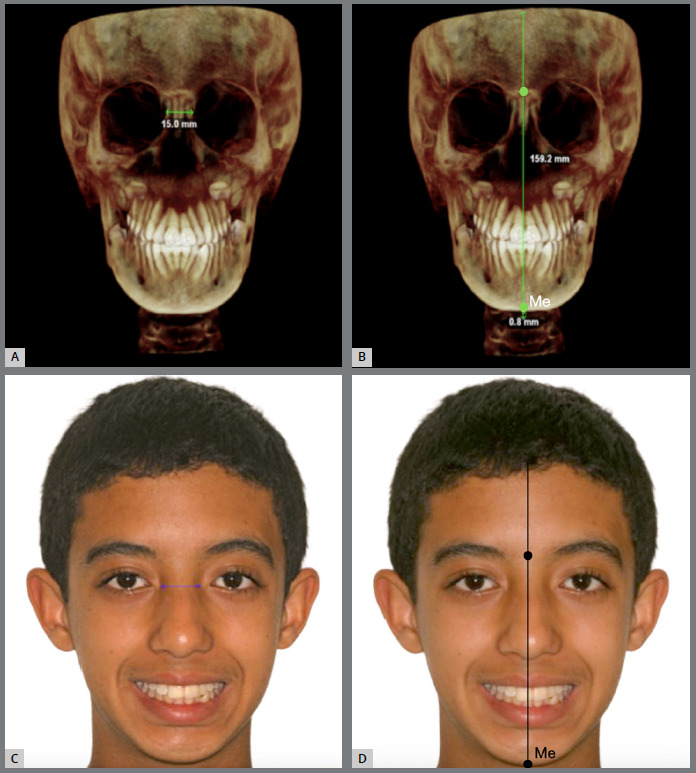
3D images obtained from CBCT scan: A) measurement of the distance between the right and left frontolacrimal sutures; B) measurement of the distance from the Me point to the VRL. Extraoral photographs: C)demonstrating the calibration of the facial photograph, wherein the distance between the frontal lacrimal sutures is transposed to the intercanthal distance on the CBCT scan, with the numerical values being identified accordingly; D) evidencing the demarcation of the facial midline. The nasion and the base of the filter (cupid’s bow at the center of the upper lip) served as soft tissue reference points for locating and determining the orientation of the facial midline.
